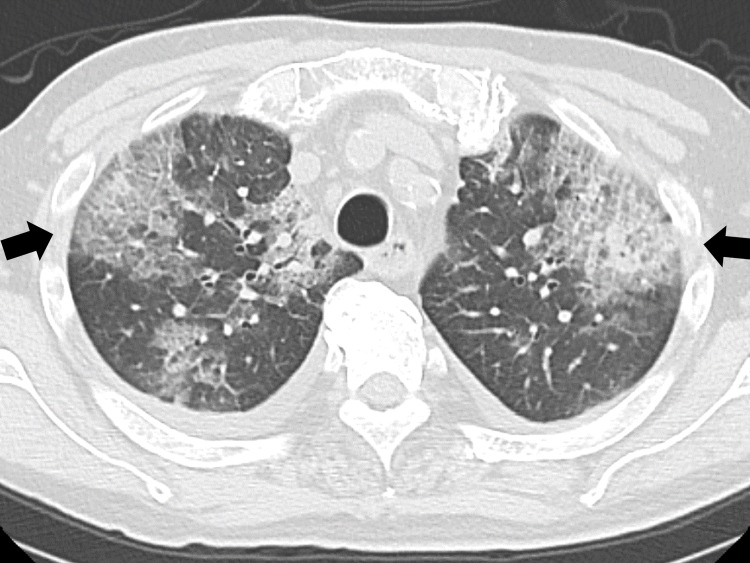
Chest CT showing bilateral pleural effusion and pan-lobular infiltration shadows (arrows) CT: computed tomography
